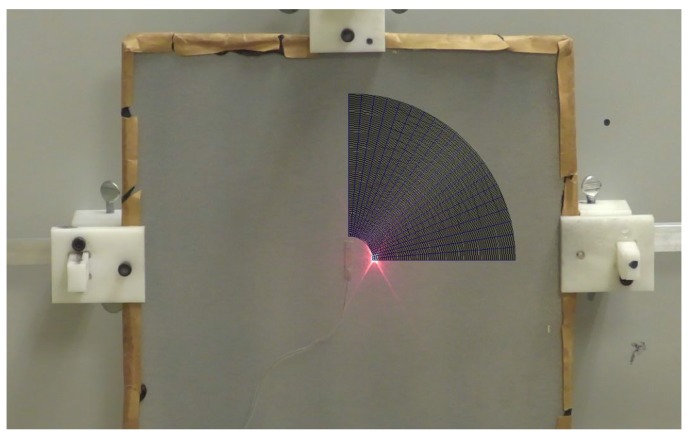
Experimental setup used to validate the 425 kHz configuration; a Polytec PSV-500-3D-M laser Doppler scanning vibrometer system was used to extract the displacement field on a circular array of points. Contacts were ensured using silver-loaded epoxy (Chemtronics Conductive Epoxy).
